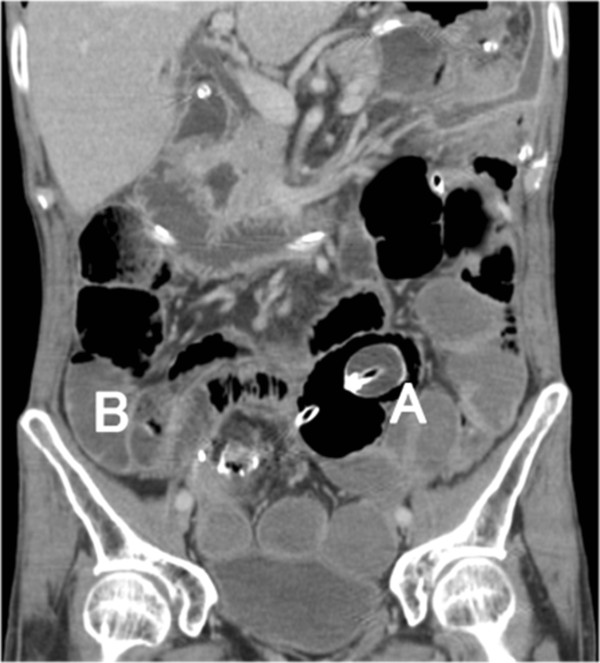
Abdominal contrast computed tomography. The tip of the ileus tube stops at the origin of the obstruction. The site of the obstruction appears to be the hernial orifice and internal herniation of the small bowel is apparent past that point. A: Ileus tube balloon, B: Internal herniation of the small bowel.
